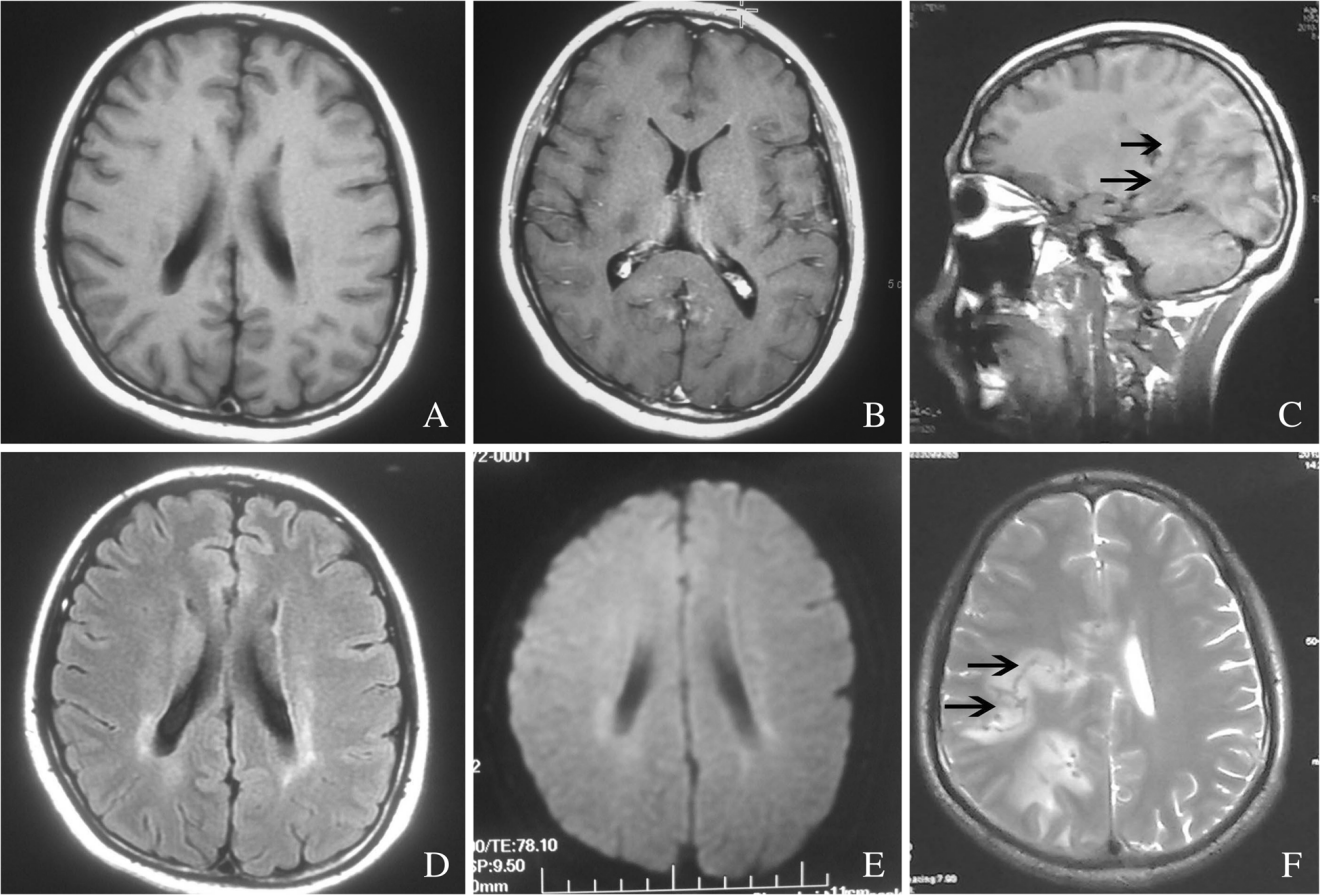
An abnormal sign on the lateral angles on the magnetic resonance imaging scan. The characteristic appearance was the ‘tunnel sign’, which was arrowed in T1 sagittal (C) and T2 axial sequences (F). FLAIR imaging (D) indicated leukoencephalopathy on the lateral angles. However, there were no remarkable changes on T1 (A), contrasted MR (B) and DWI imaging (E).
